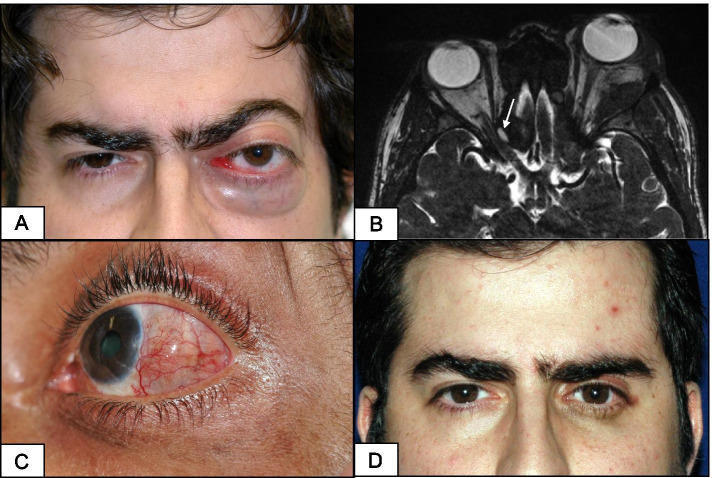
A External photo of a male patient (case 3) presenting with a left orbital RDD and a significant left axial proptosis. B Axial high-resolution MRI showing bilateral orbital infiltration with right intracanalicular optic nerve encroachment (arrow) in case 2. C External photo of a patient (case 5) with scleral RDD showing inflamed and edematous sclera with conjunctival, superficial and deep episcleral vascular congestion. D Excellent result was observed in case 3 shown in A
